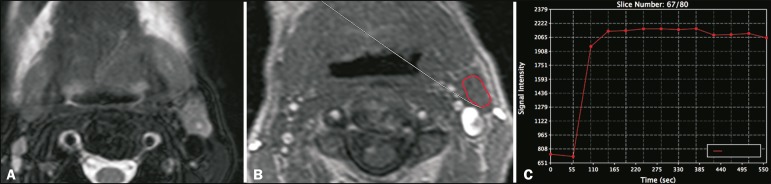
DCE MRI scan of a 72-year-old male patient with left oral tongue
